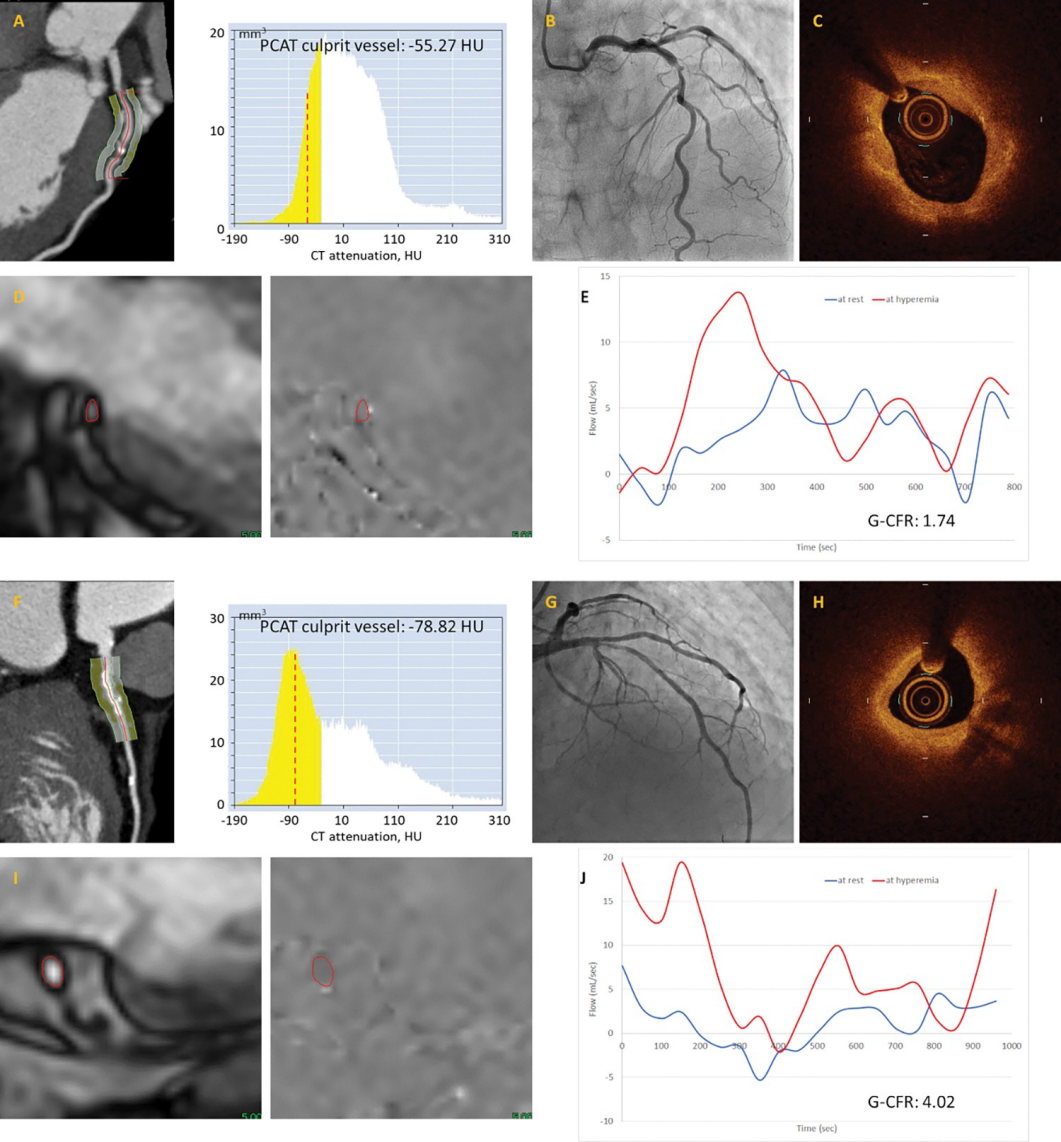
Representative images of patients with and without layered plaque. (Upper panel) A case of non-ST-segment-elevation acute coronary syndrome (NSTE-ACS) with layered plaque. (A) Coronary computed tomography angiography (CCTA) revealed that the pericoronary adipose tissue (PCAT) attenuation value of the left anterior descending artery (LAD) and that of the three-vessel-PCAT were -55.27 HU and -60.14 HU, respectively. (B) Coronary angiography revealed a stenosis in the proximal LAD. (C) Optical coherence tomography (OCT) revealed the presence of layered plaque. (D, E) Phase contrast (PC) cine-cardiac magnetic resonance imaging (CMR) of the coronary sinus measurement revealed that the global coronary flow reserve (G-CFR) was 1.74. (Lower panel) A case of NSTE-ACS with non-layered plaque. (F) CCTA revealed that the PCAT attenuation value of the LAD and that of the three-vessel-PCAT were -78.82 HU and -75.12 HU, respectively. (G) Coronary angiography revealed a stenosis in the proximal LAD. (H) OCT revealed the absence of layered plaque. (I, J) PC-CMR revealed that the G-CFR was 4.02.
